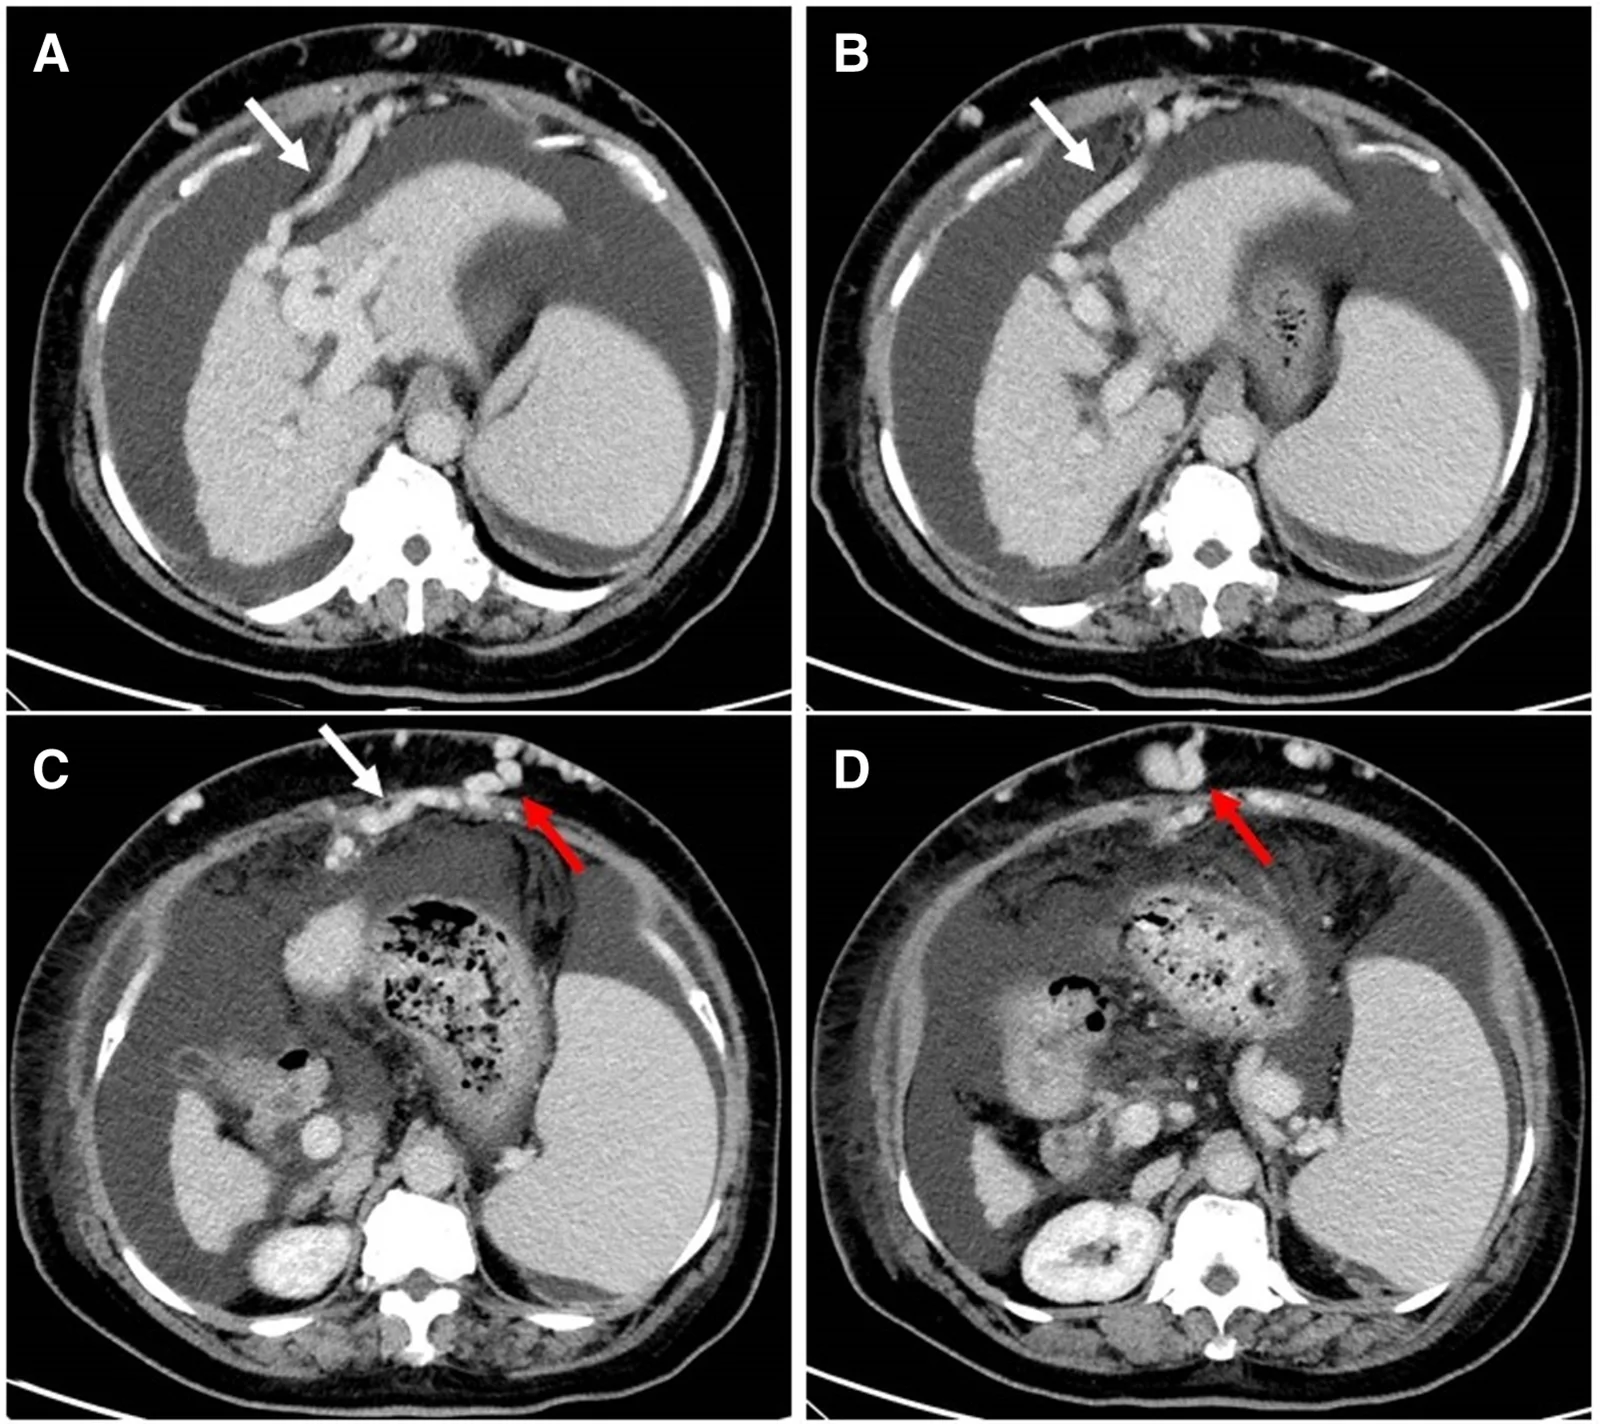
Abdominal contrast-enhanced CT showed umbilical vein recanalization with abdominal wall varicosis. The white arrow refers to umbilical vein recanalization with a diameter of approximately 7.3 mm. The red arrow refers to abdominal wall varicosis.
